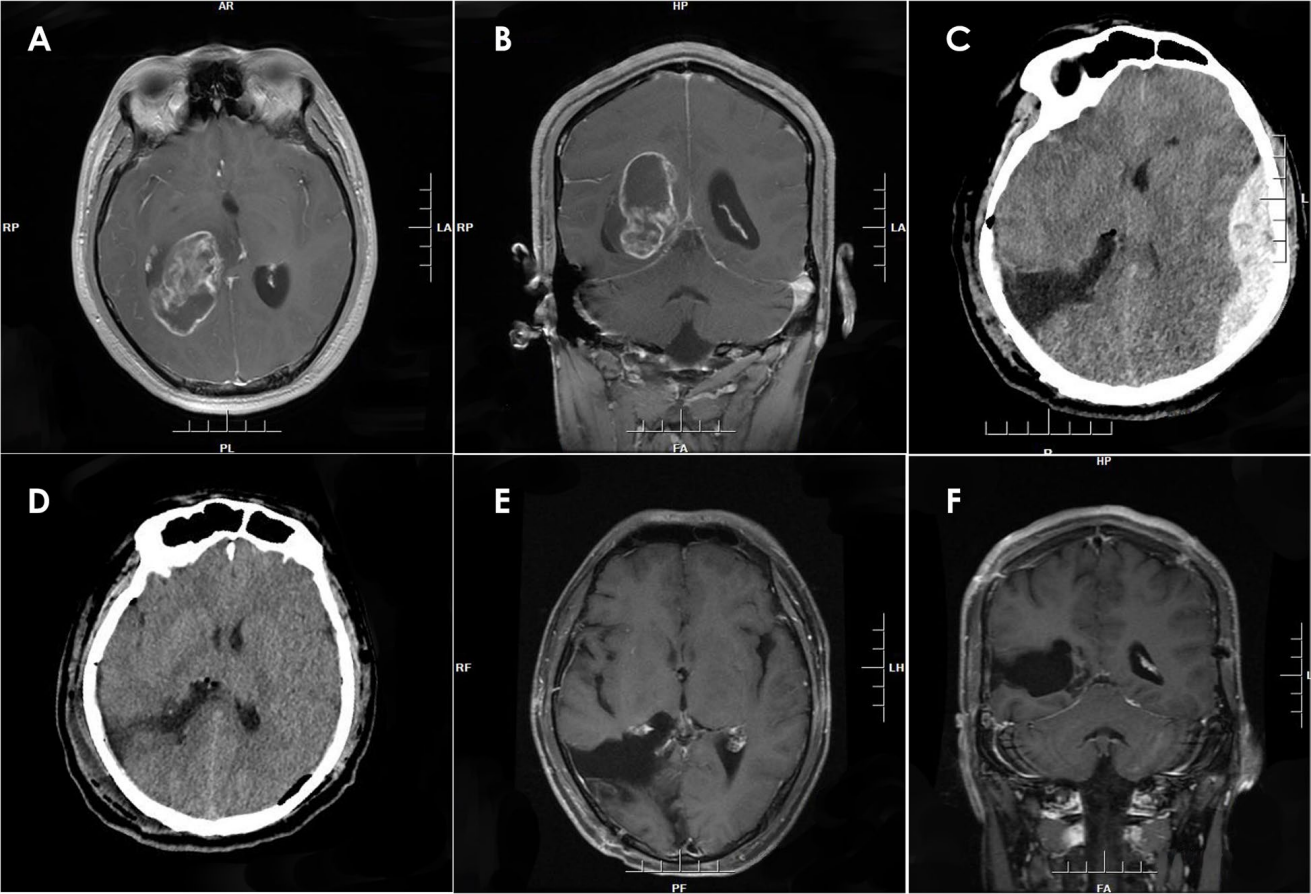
The enhanced MRI showed a tumor in the right ventricle (A, B). The postoperative CT showed PEDH on the contralateral (left) temporal-parietal-occipital region (C). CT of the patient following acute extradural hematoma evacuation surgery (D). And enhanced MRI (E, F) 3 months post-surgery was obtained for a follow-up examination
